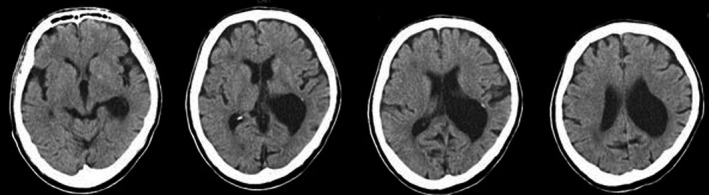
Brain computed tomography (CT) scans immediately after the hypoxic insult.
